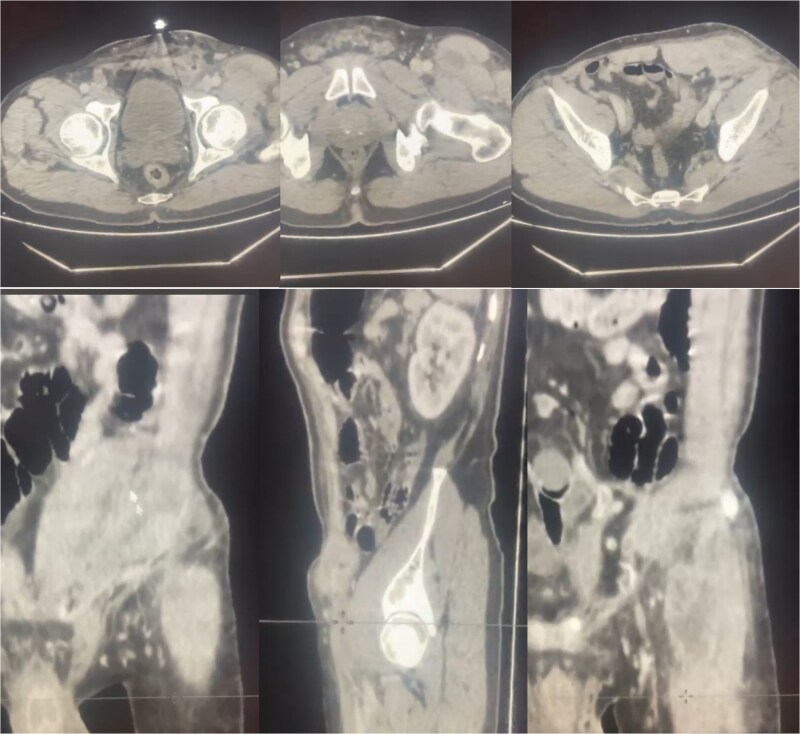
Abdominal pelvic CT-scan showing inflammation around the left inguinal mesh with infiltration and thickening around the adjacent parietal soft tissues and multiple collections surrounding the mesh and along the ipsilateral iliopsoas and sartorius muscles.
